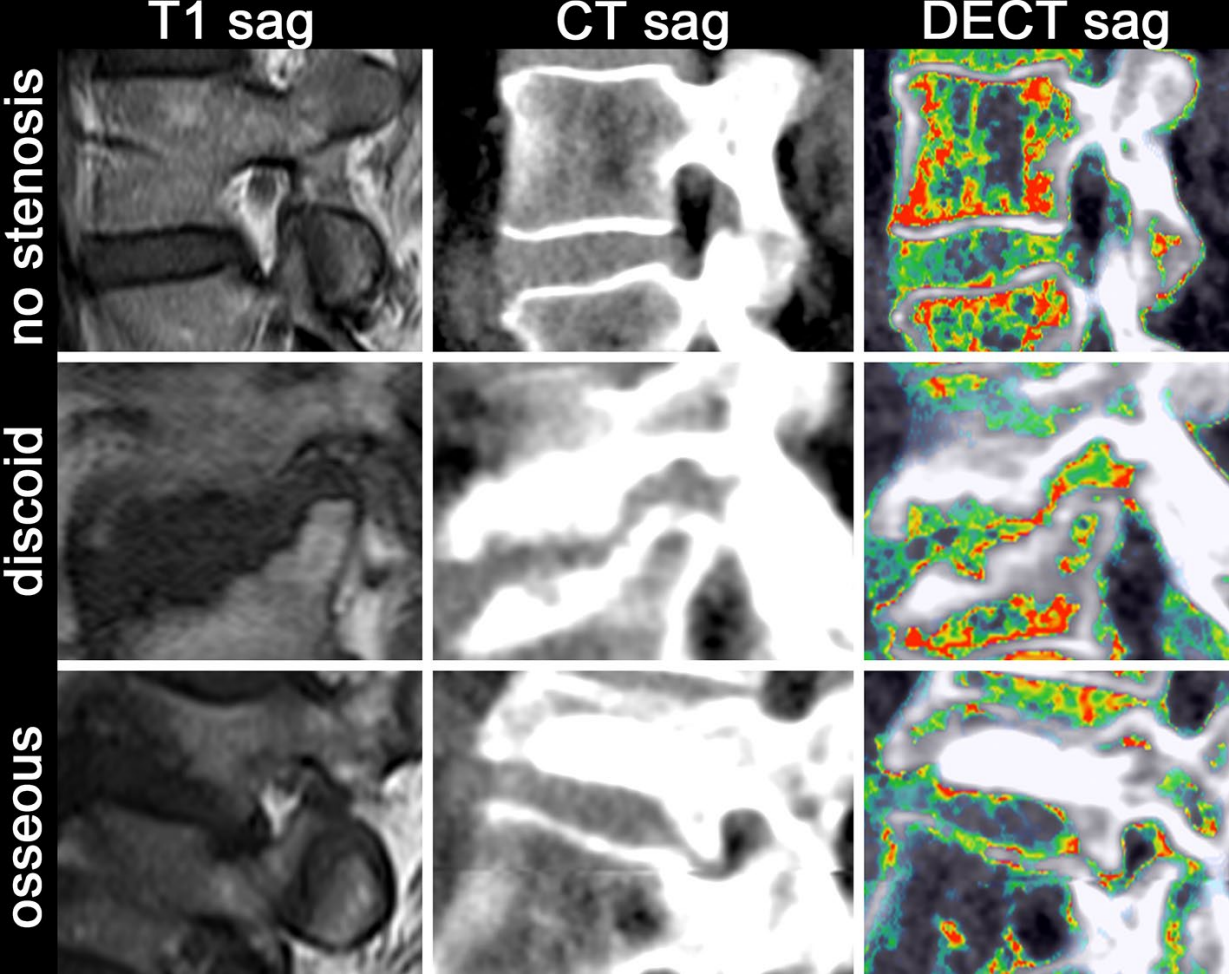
Imaging examples of no foraminal stenosis as well as discoid and osseous stenosis in (left to right) MRI, standard CT, and collagen-sensitive dual-energy reconstructions.
